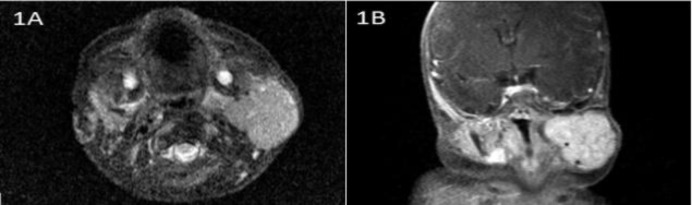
a) Neck MRI, T2-weighted axial image; b) contrast enhanced image showing left-sided hyperintense mass lesion with contrast enhancement
